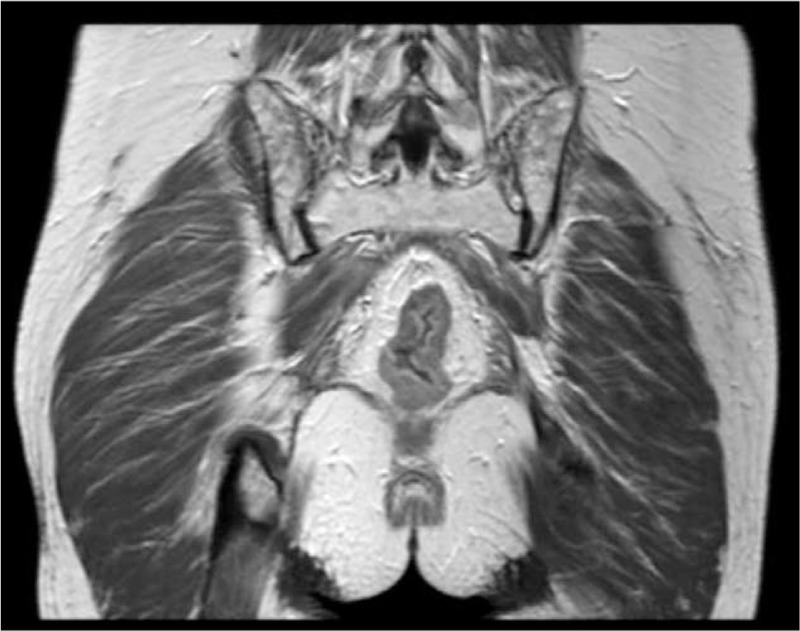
Magnetic resonance imaging examination of the patient before the treatment showed thickening and swelling of the colorectal wall.
